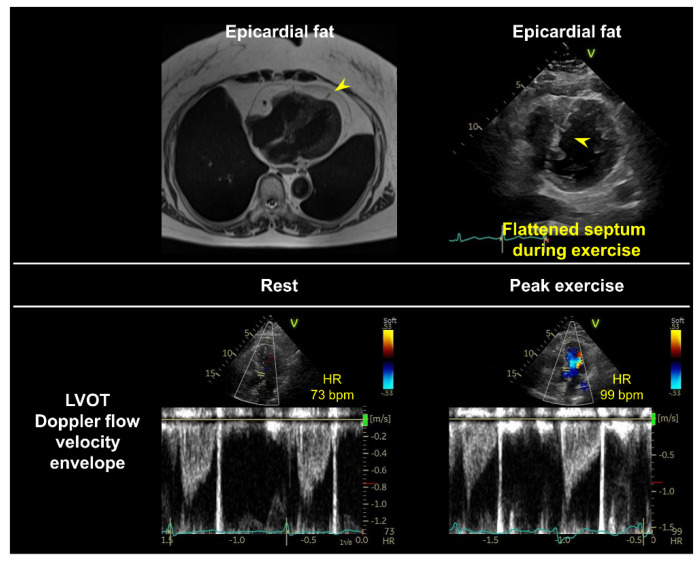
A case of cardiac output (CO) reserve limitation during exercise. A 79-year-old woman with obesity (body mass index, 30 kg/m^2^) was referred to our echocardiographic laboratory for the evaluation of unexplained dyspnea on exertion. Her NP levels were within the normal range (N-terminal pro-brain NP, 65 pg/mL). The results of resting echocardiography were also normal; however, cardiac magnetic resonance imaging showed remarkable epicardial fat tissue (yellow arrowhead). Diastolic stress echocardiography combined with expired gas analysis showed CO reserve limitation during exercise relative to increases in VO_2_ (CO, 2.9 to 3.9 L/min; VO_2_, 189 to 549 mL/min; CO reserve, 48%). The septum became flattened in the parasternal short-axis view during peak ergometry exercise (yellow arrowhead), suggesting that enhanced ventricular interdependence might have contributed to the CO reserve limitation due to reduction in LV preload in addition to chronotropic incompetence. LVOT, left ventricular outflow tract.
